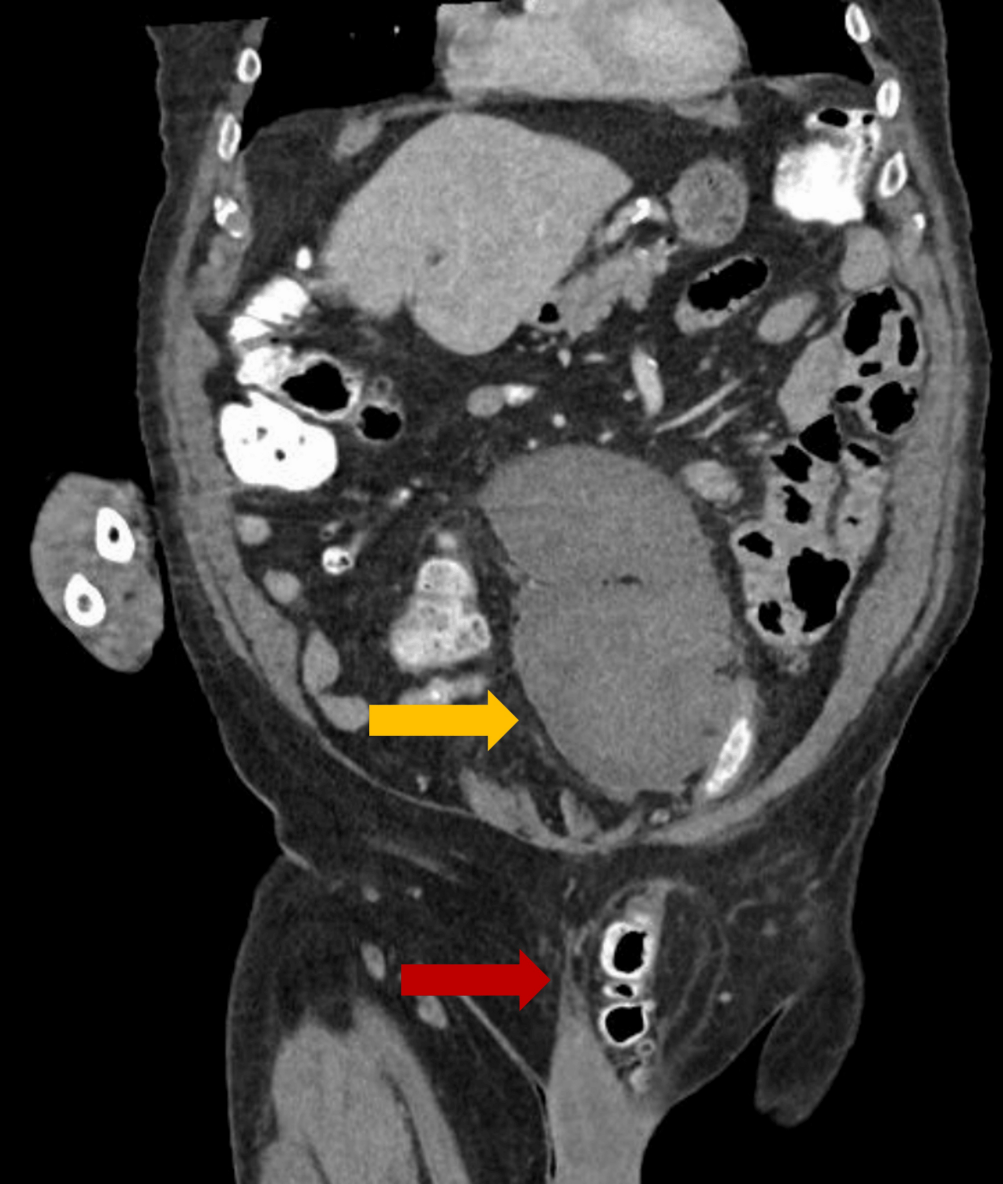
Abdominal Computed Tomography Scan of the Sigmoid Colon Hernia and Mesenteric Hematoma Orange arrow: mesenteric hematoma; red arrow: sigmoid colon hernia
